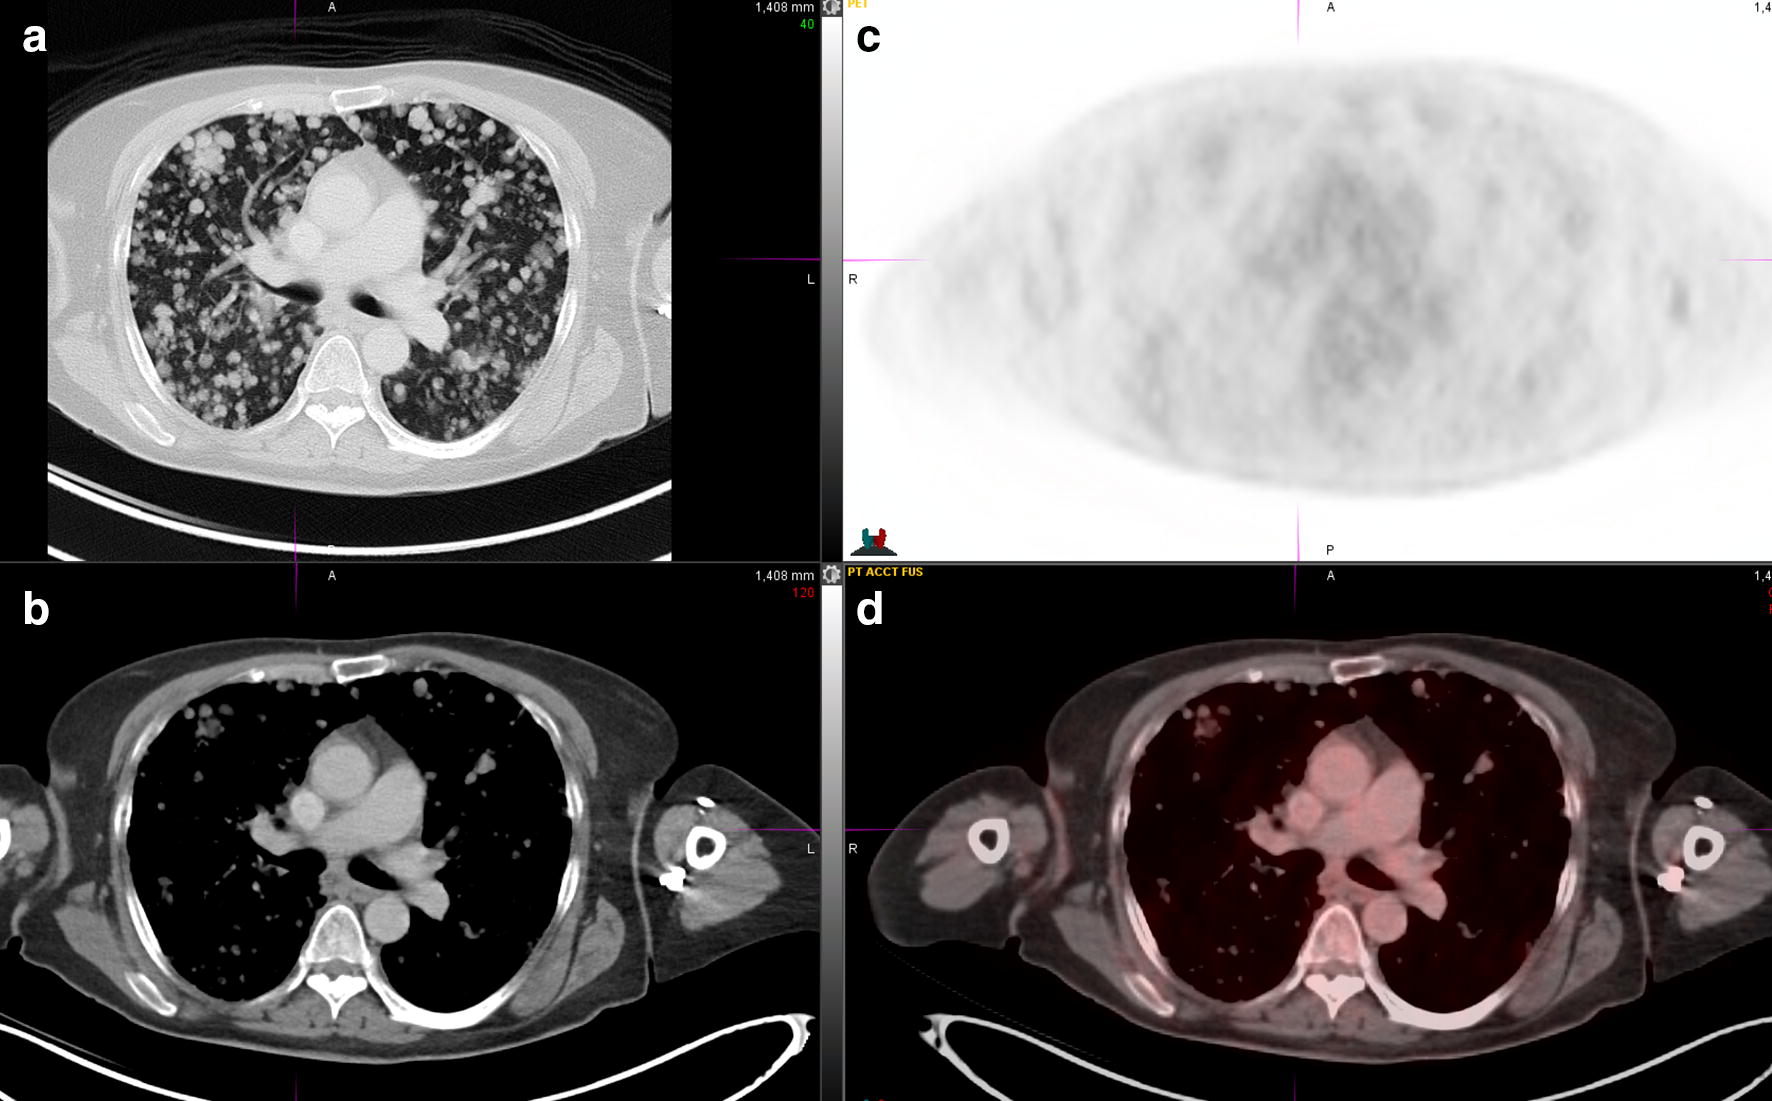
PET/CT 4 years since pazopanib was started. a CT lung window; b CT mediastinal window; c PET; d fusion CT and PET
